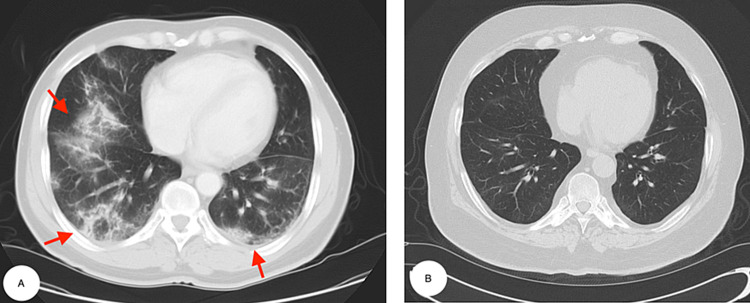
CT scan of the chest before treatment and after two years on treatment. (A) Patchy bilateral ground-glass opacities and infiltrates (red arrows). (B) Resolution of the infiltrates.
